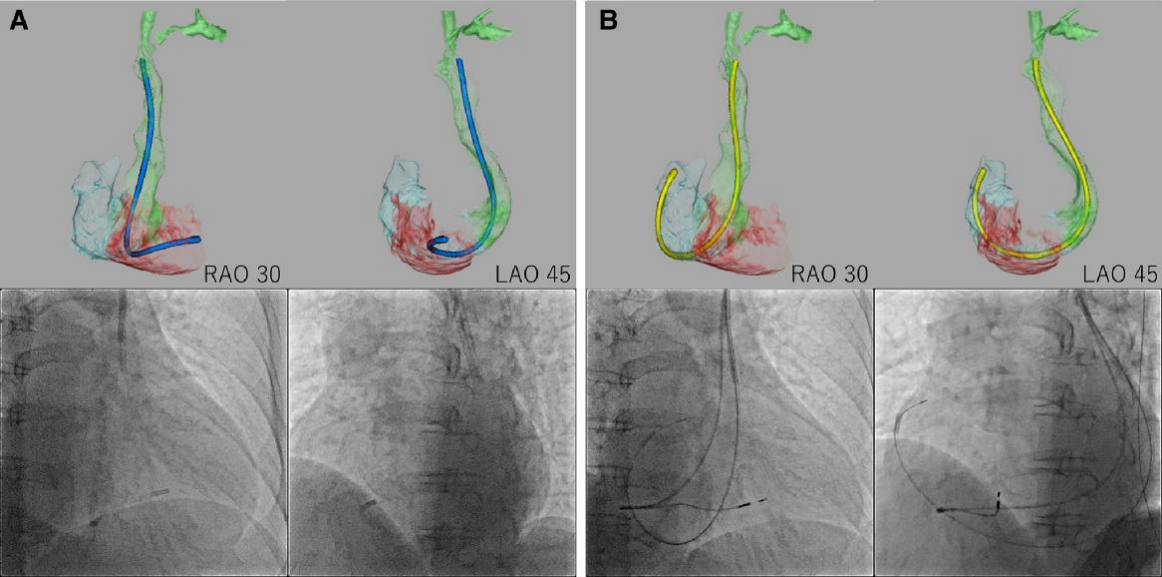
Delivery catheter merged with cardiac multidetector computed tomography. We imported the delivery catheter data and reconstructed them into 3D computed tomography, then merged them with the patient’s cardiac 3D multidetector computed tomography. (*A*) The distal end of the C315-S10 delivery catheter was in a similar position relative to the right ventricular septum between the 3D CT image and the fluoroscopic image. (*B*) The distal end of the C315-H40 delivery catheter was in a similar position relative to the right atrial appendage between the 3D computed tomography image and fluoroscopic image.
